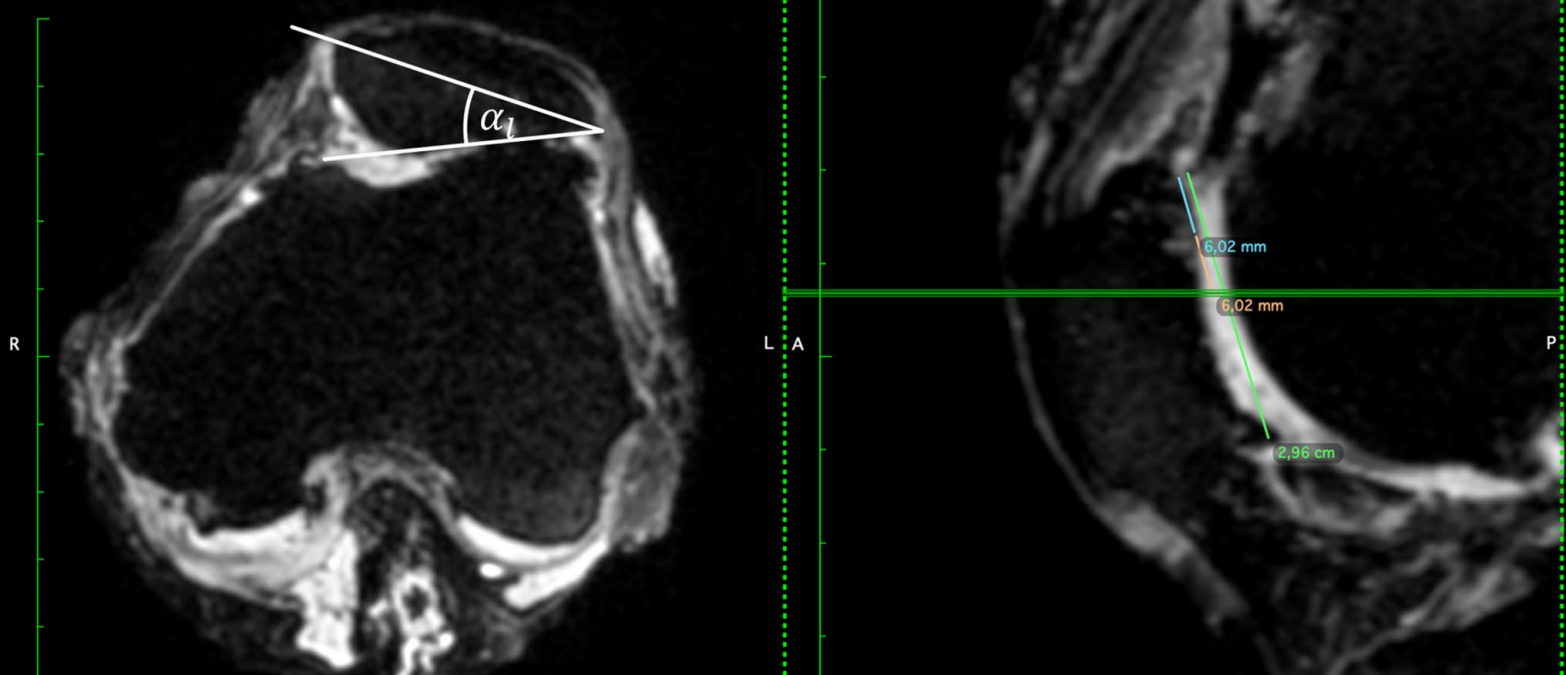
Visualization of the angles measured in the MRI data of the native knee joint. Left: patello-femoral joint in view from distal with measured lateral patellar facet angle. Right: sagittal view with the actual plane for the distal view (green). The angle drawn in the left image was measured in four parallel and equidistant planes of the patella.
